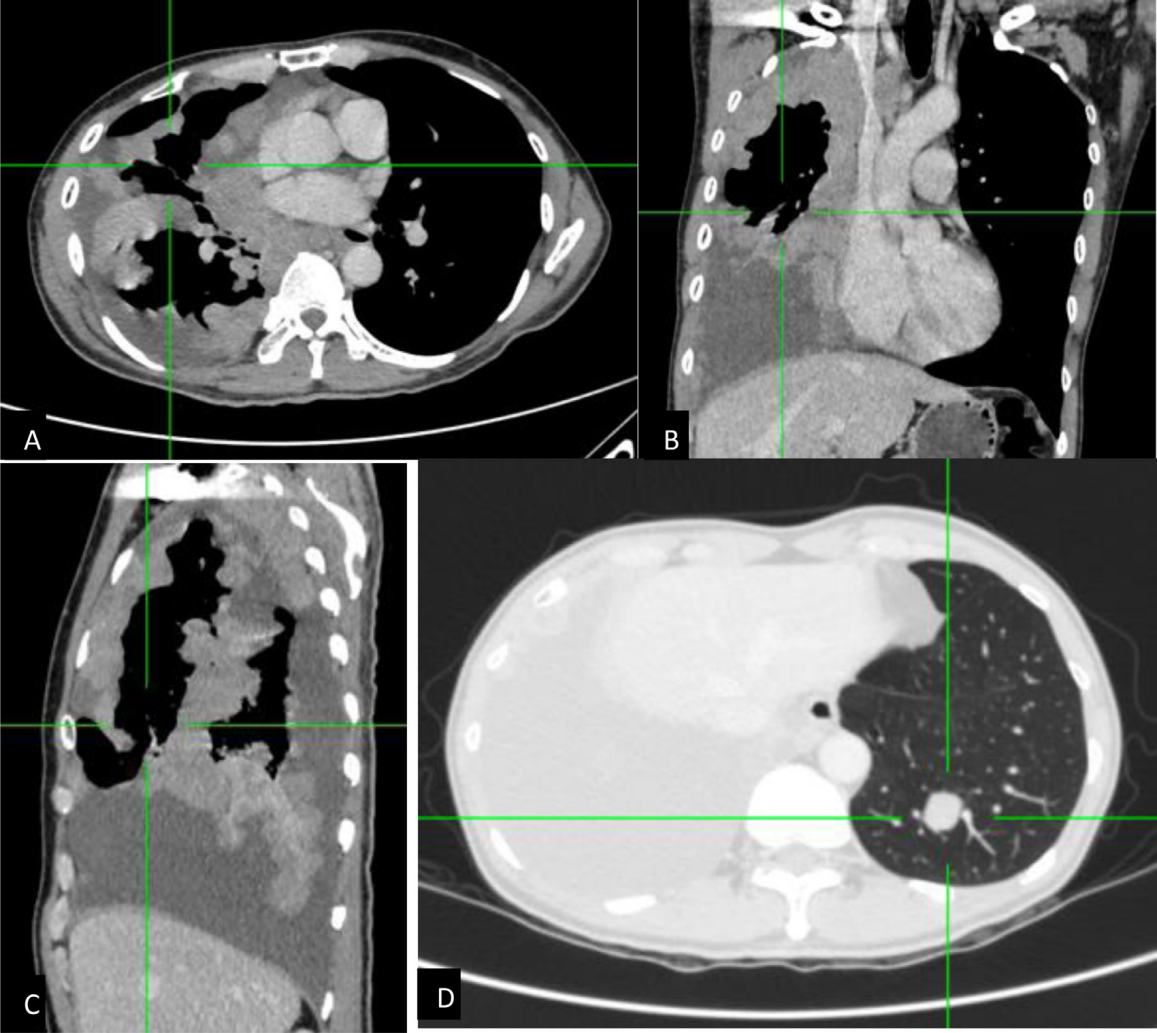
Axial (A) with coronal- (B) and sagittal-reformatted (C) contrast-enhanced chest CT showed diffuse irregular pleural thickening involving fissures, parietal, visceral, mediastinal, and diaphragmatic pleura. Multiple nodules in the left lung were confirmed, with the largest dimension measuring approximately 1.9 cm (D).
